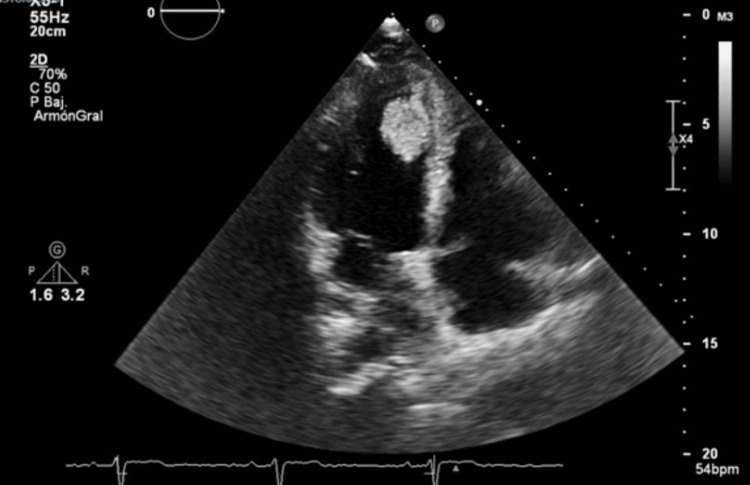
Apical four-chamber view showing a mobile intracavitary mass within the left ventricle.
